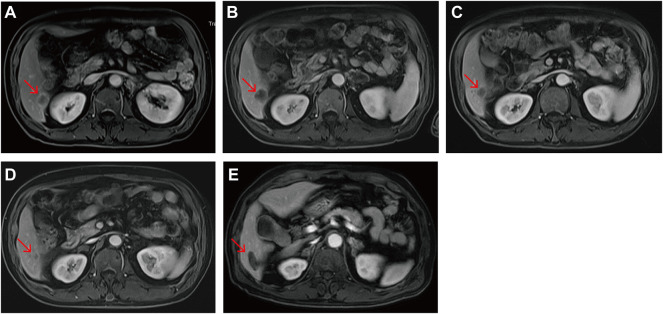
Liver metastasis magnetic resonance images of the POLE-mutant rectal cancer patient receiving camrelizumab and anlotinib. (A) Pre-immunotherapy; (B) 1 month post—immunotherapy; (C) 3 months post—immunotherapy; (D) 7 months post—immunotherapy; (E) 9 months post—immunotherapy. The image of the metastasis 2 months after argon-helium cryoablation. Red arrows indicate the same liver metastases.
